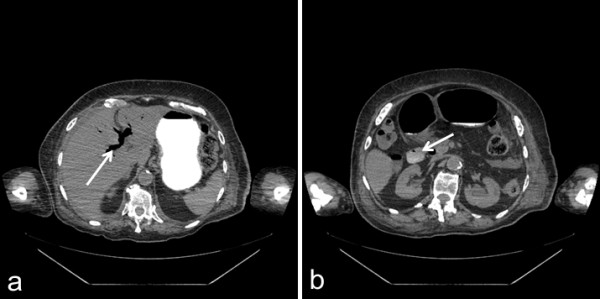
Computed tomography image (axial view) showing Rigler’s triad. a Gastric distension and pneumobilia (arrow). b Gallstone in the duodenal bulb (arrow).
